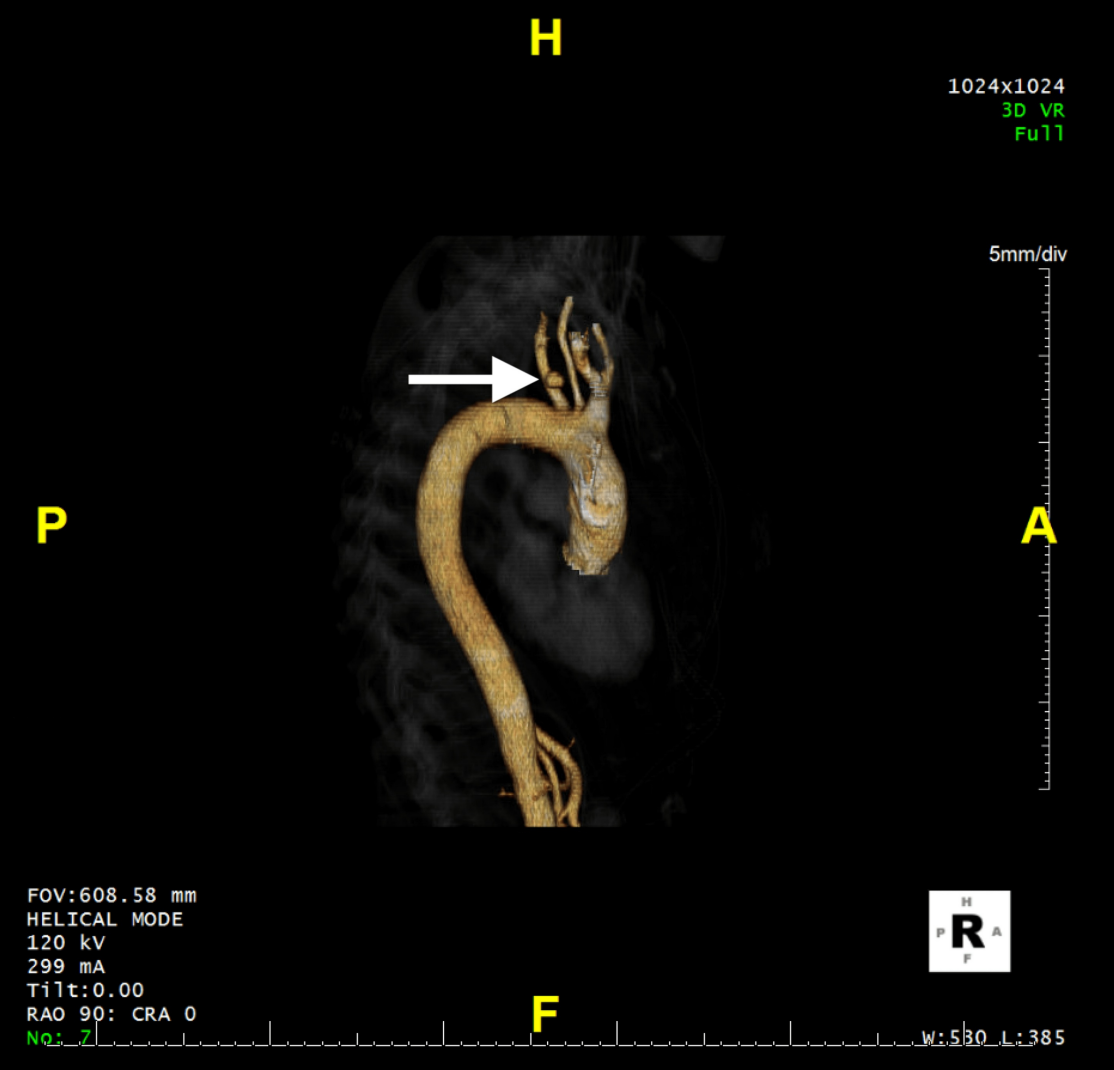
Left subclavian artery pseudoaneurysm visualized on three-dimensional (3-D) CT angiography. 3-D, volume-rendered CT angiogram demonstrating a pseudoaneurysm arising from the proximal left subclavian artery (white arrow), adjacent to the aortic arch.
